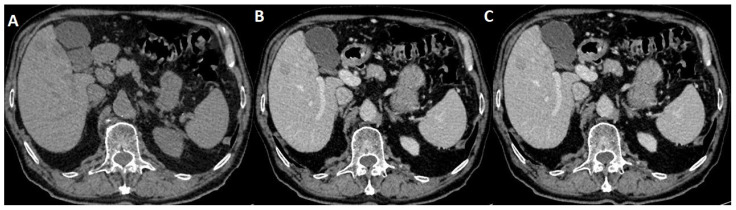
(A) Native transversal CT incidental findings on hepatic structure with multiple masses; (B) arterial iodate CT scanning showing hypoattenuating masses; (C) venous CT iodate appearance of nodular enhancement.
